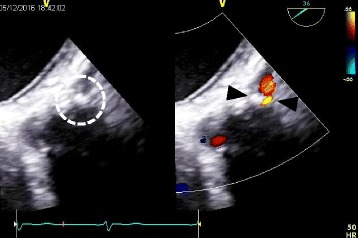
Intraoperative transesophageal echocardiography. *Left*, a high-brightness massive shadow at the left coronary ostium (*dotted circle*). *Right*, color flow on Doppler showed a mosaic image and accelerated blood flow (*arrowhead*)
